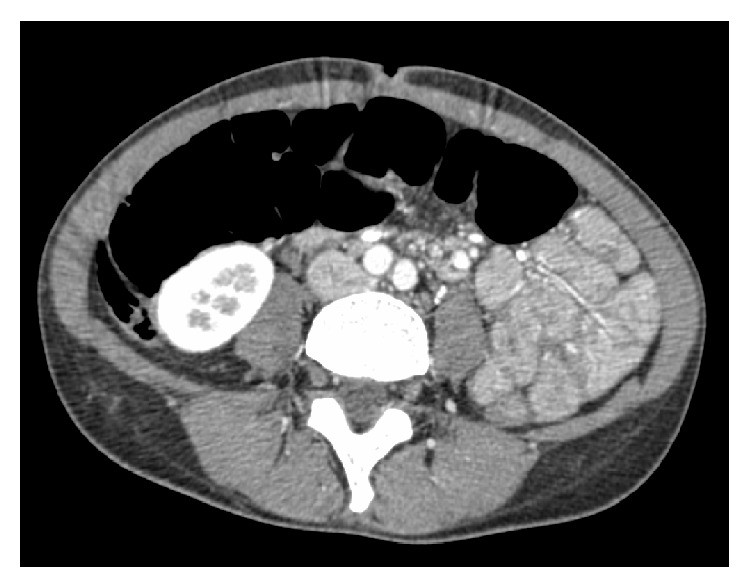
Contrast-enhanced abdominal computed tomography demonstrating small intestine wall thickening with fluid retention.
